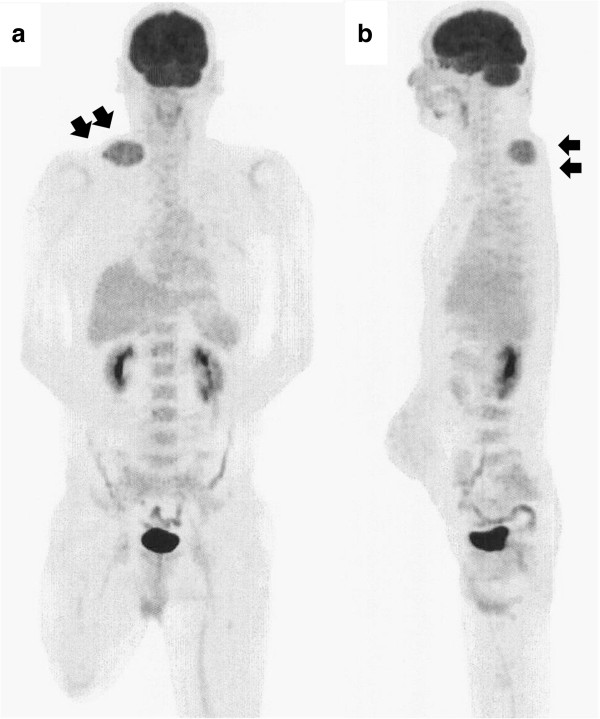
FDG uptake in the trapezius muscle but not in the pulmonary metastasis. (a) Anteroposterior and (b) lateral maximum intensity projection PET image demonstrates slight heterogeneous FDG uptake in the tumor located in the trapezius muscle (SUV = 4.6). Accumulation of the tracer in the pulmonary metastases was insignificant, and accumulation suggestive of metastasis was not found on the PET-CT scan, with the exception of the trapezius muscle.
